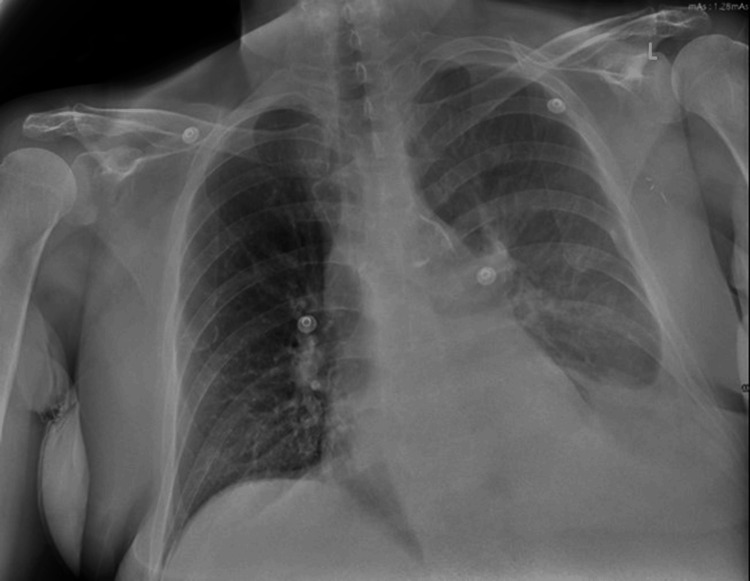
Chest X-ray showing left lower lobe opacity and blunting of the costophrenic angle consistent with pleural effusion
